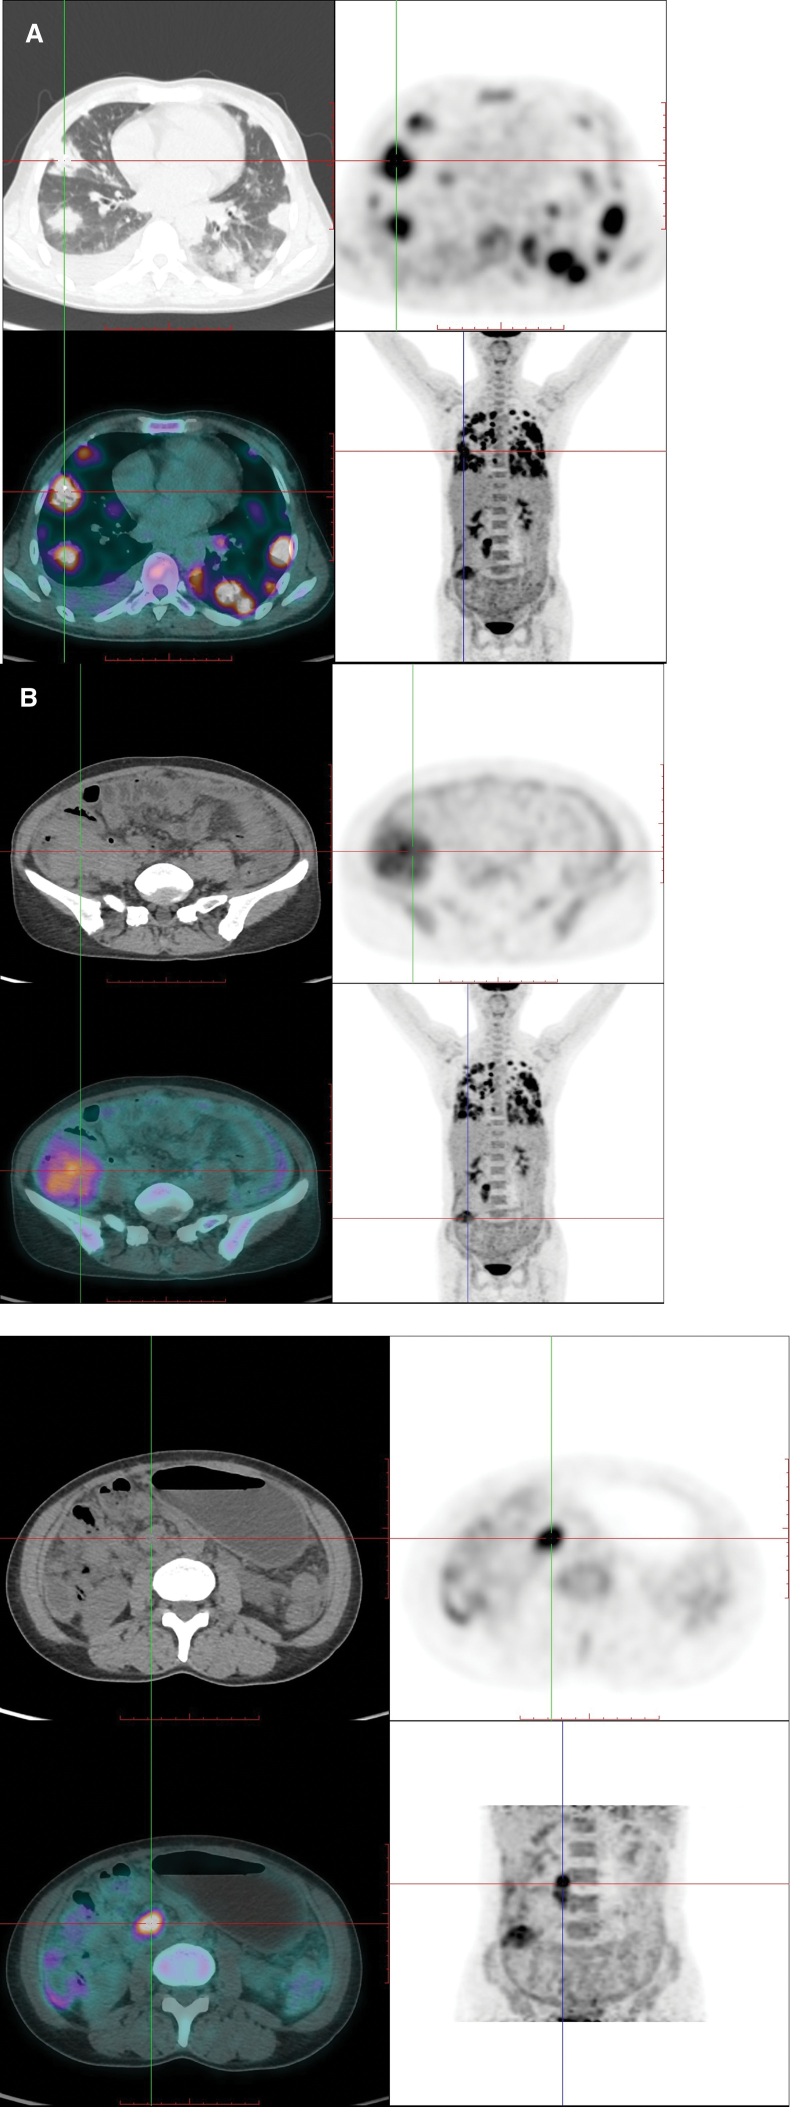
(A) The whole-body PET/CT images showed multiple nodular and patchy density-increasing lesions with significantly increased FDG uptake in both lungs. (B) Soft tissue mass with increased FDG uptake in the lower ascending colon. (C) Retroperitoneal lymph node enlargement with increased FDG uptake on delayed imaging. FDG = fluorodeoxyglucose, PET/CT = positron emission tomography/computed tomography.
